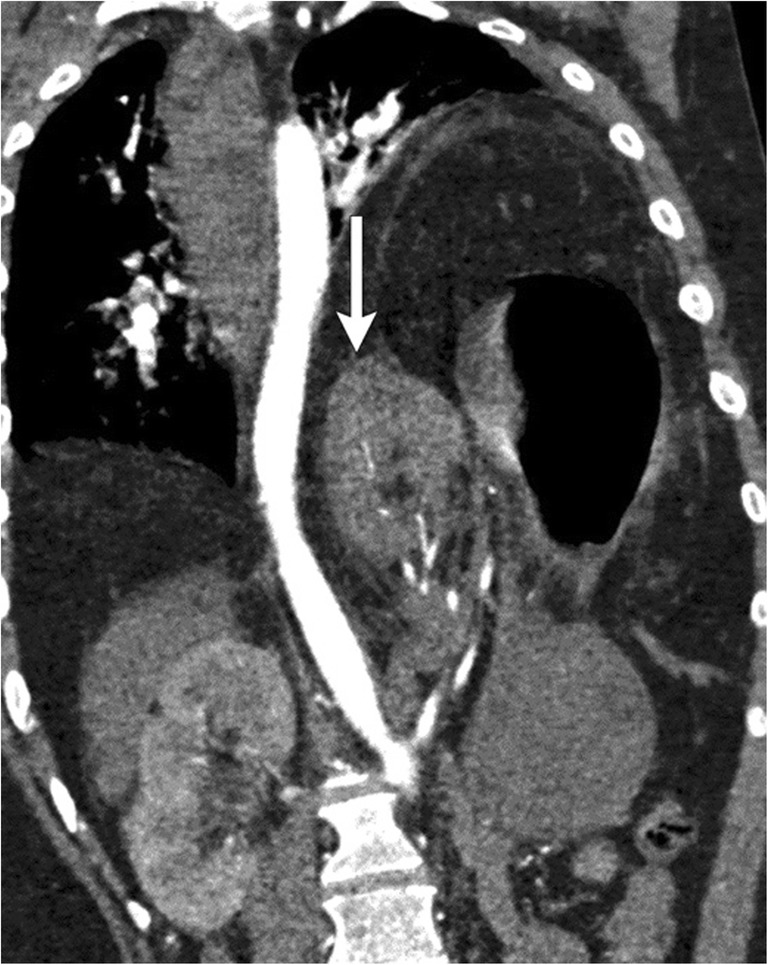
A 31-year-old patient who was on bed rest for lower extremity fracture, now presenting with new-onset chest pain following a severe bout of coughing. Coronal contrast CT demonstrates a large left diaphragmatic hernia, with left kidney, stomach and omentum present within the left thorax. Note decreased enhancement of the left kidney (white arrow) compared with the right kidney on these arterial phase images. The renal artery is normal; the renal vein cannot be evaluated on these arterial-phase images. Intraoperative findings were consistent with these findings demonstrating narrowing of the left renal vein with venous engorgement at the level of the diaphragmatic defect (Movie 5)
